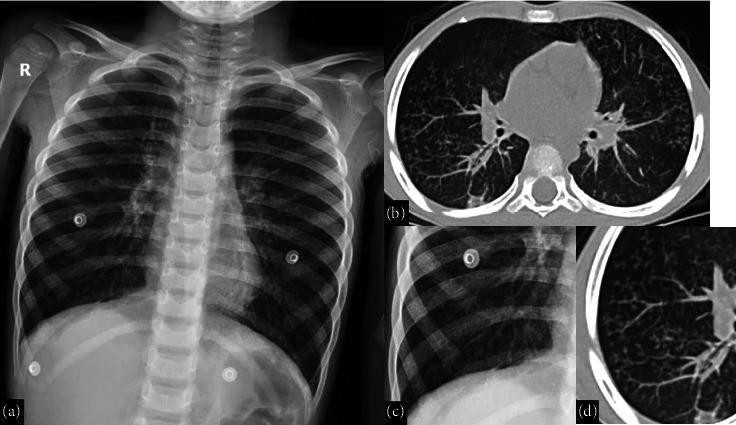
A 6-year-old male with a history of bronchial asthma who acquired COVID-19 infection. He was presented with fever and cough. (a) Chest X-ray with a magnified view (c) shows faint reticulonodular opacities. (b) Axial CT of the chest (lung window) with a magnified view (d) shows centrilobular nodules with opacified distal bronchioles (tree-in-bud sign), which is an atypical finding for pediatric COVID-19 pneumonia. The patient clinically improved and was discharged after one week.
